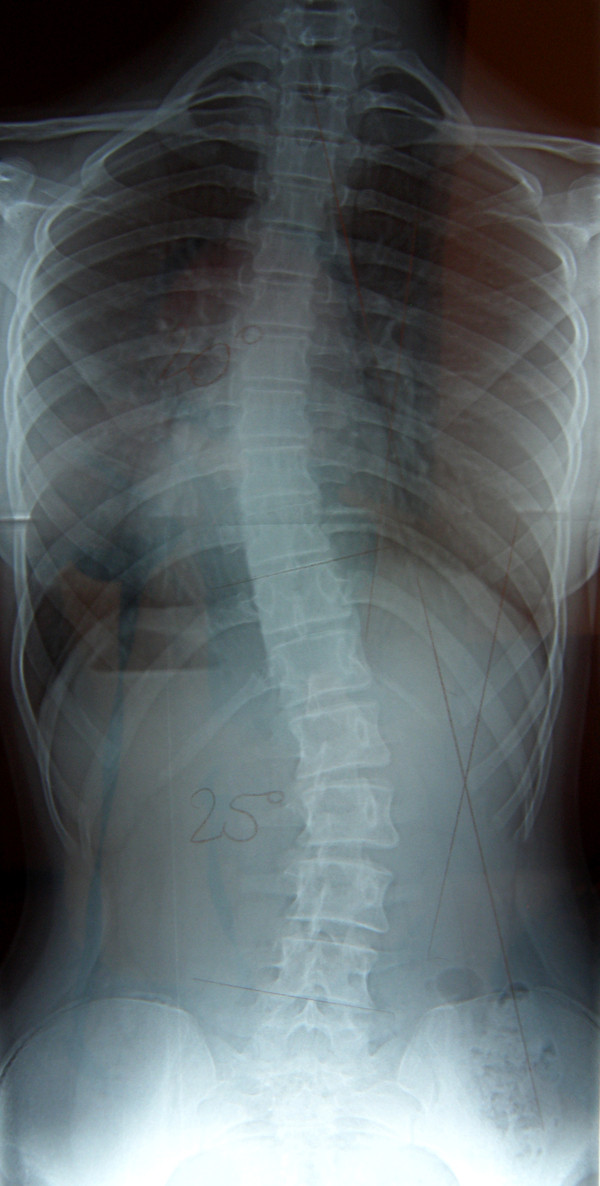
Radiography after treatment.
